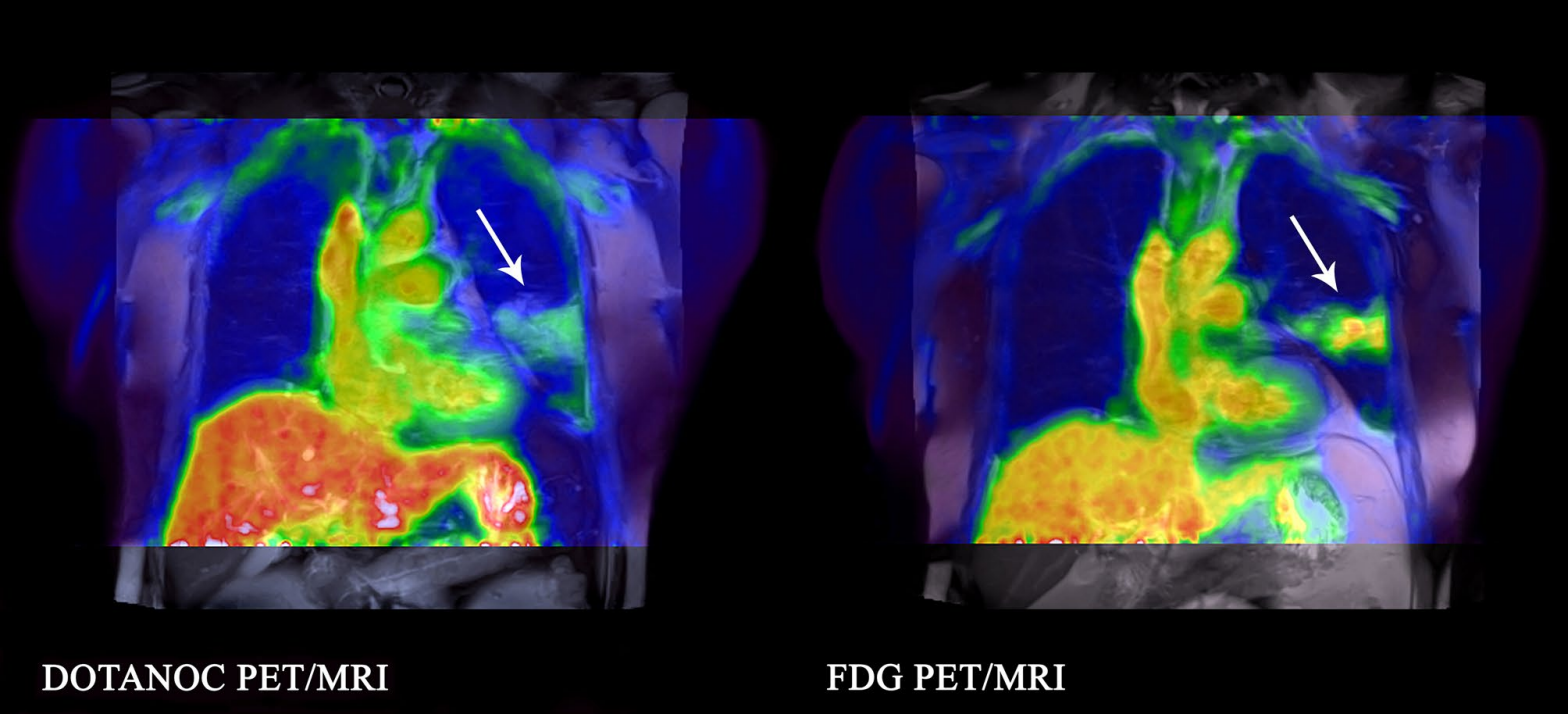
Similar distribution of tracer uptake of DOTANOC and FDG in a 73-year old male patient with non-TB pneumonia. Lesion visualised in the left lower lobe mainly involving the superior segment.
